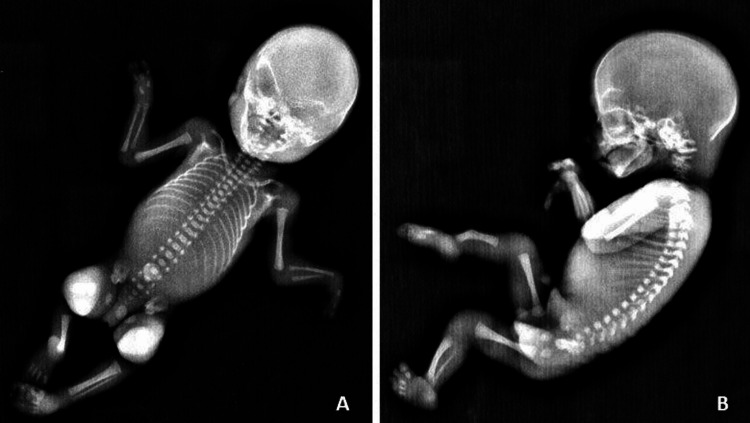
(A) Fetal X-ray showing bilateral hand malformations and left ulnae hypoplasia; (B) Radiograph of lower limbs showing right fibular aplasia and tibial campomelia with missing metatarsal bones and digits bilaterally.
